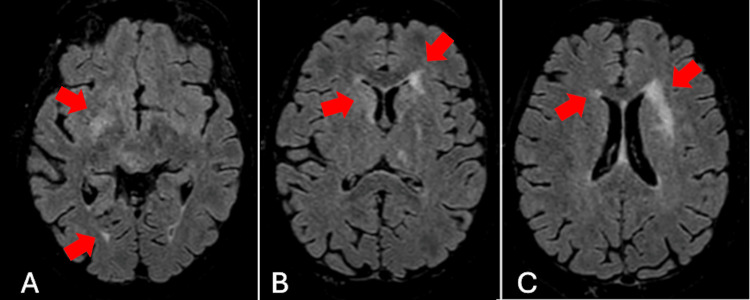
Axial T2/FLAIR MRI demonstrating asymmetrical subcortical (A) and periventricular hyperintensities (B-C) involving the deep white and grey matter at initial presentation, in the context of subacute encephalopathy. Abbreviations: FLAIR=fluid-attenuated inversion recovery; MRI=magnetic resonance imaging
